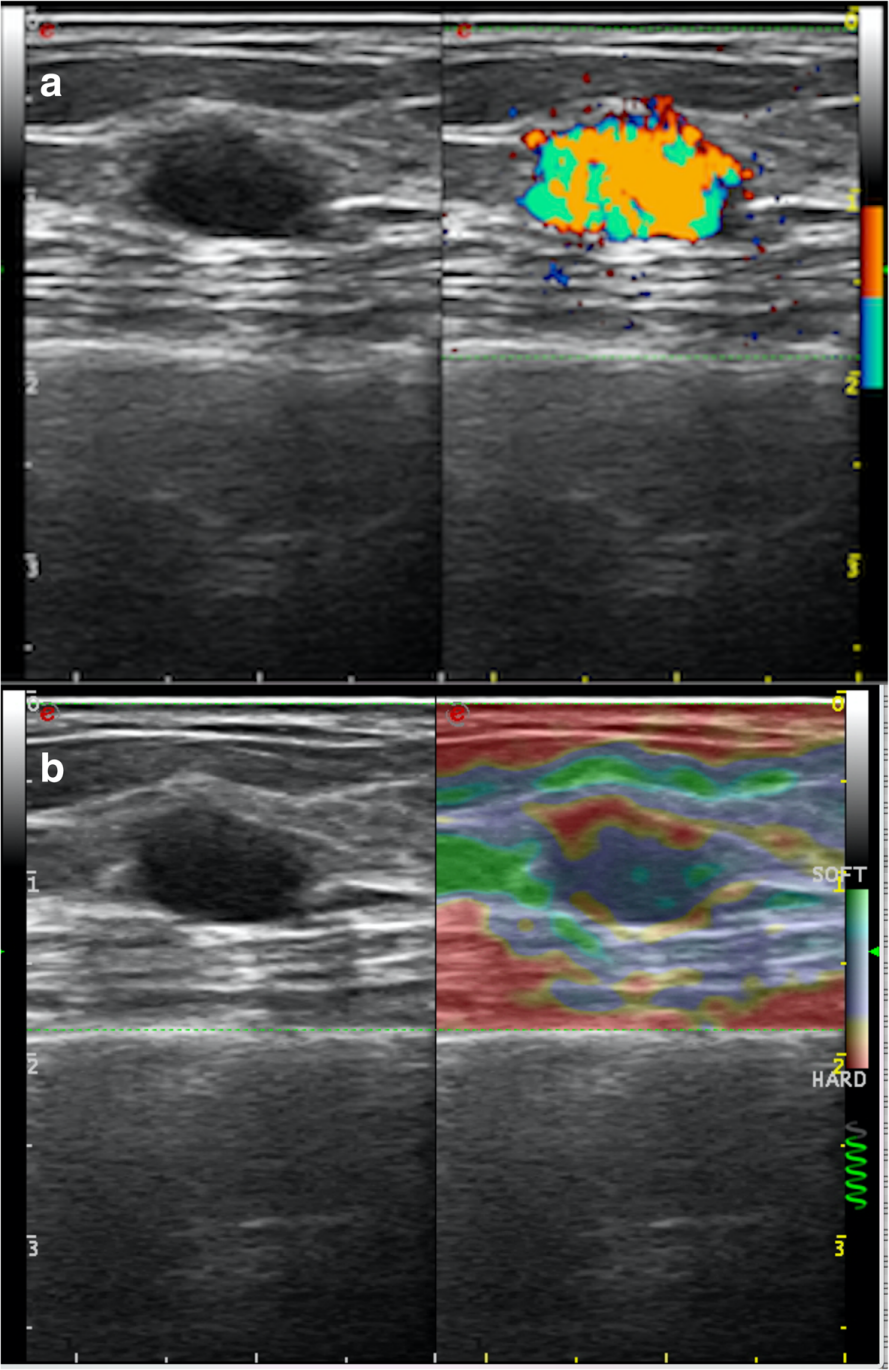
Breast ultrasonography. Ultrasound of the breast showed an oval, hypo-echoic nodule with well-defined margins and no shadowing. Extensive vascularity was also noted. The RI was 0.62–0.67 (a). Elastography revealed an E2 lesion (b)
